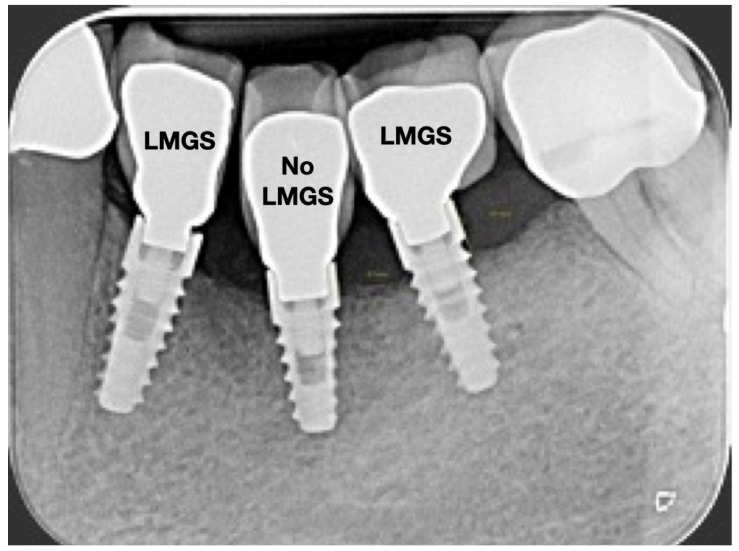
Radiograph of the same three (3) implants at 10 years. (LMGS = with a laser-microgrooved collar surface; No-LMGS = without a laser-microgrooved collar surface).
